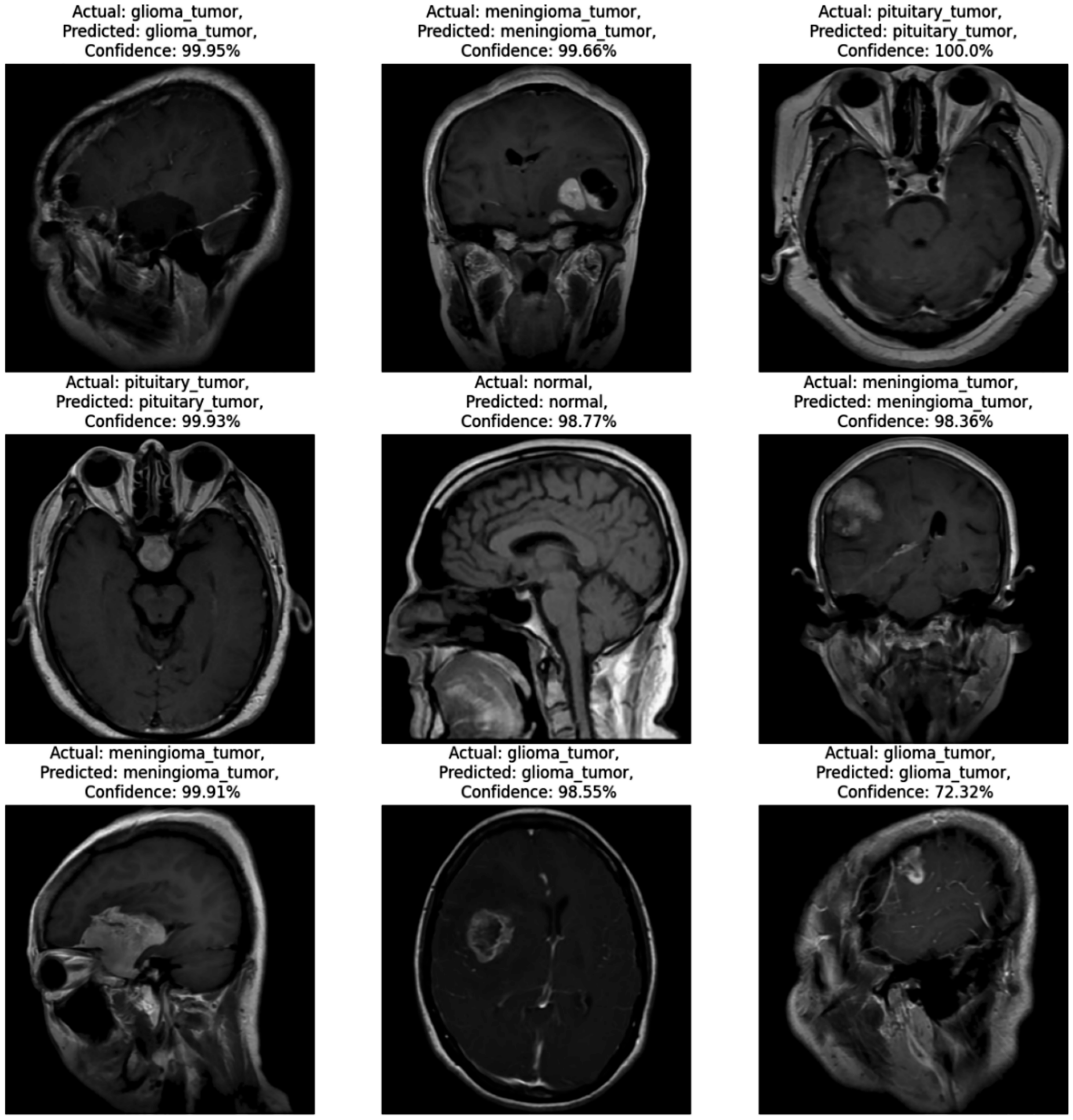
Example MRI predictions: actual vs. predicted tumor types with model confidence levels.
